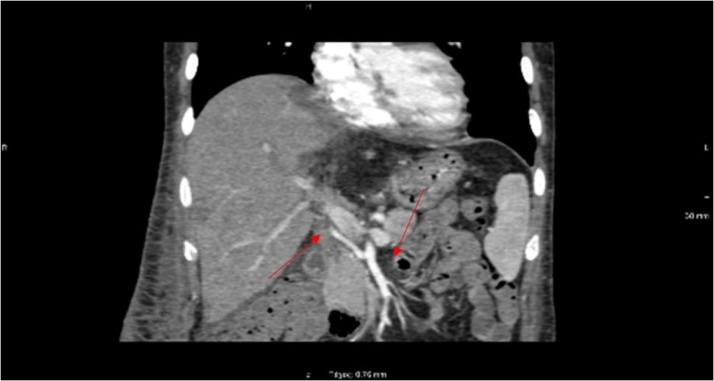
CTA of the abdominal aorta; Oblique maximum intensity projection image demonstrates the hepatic artery originating from the superior mesenteric artery. It also shows filling defect of the distal hepatic artery, followed by total occlusion.
